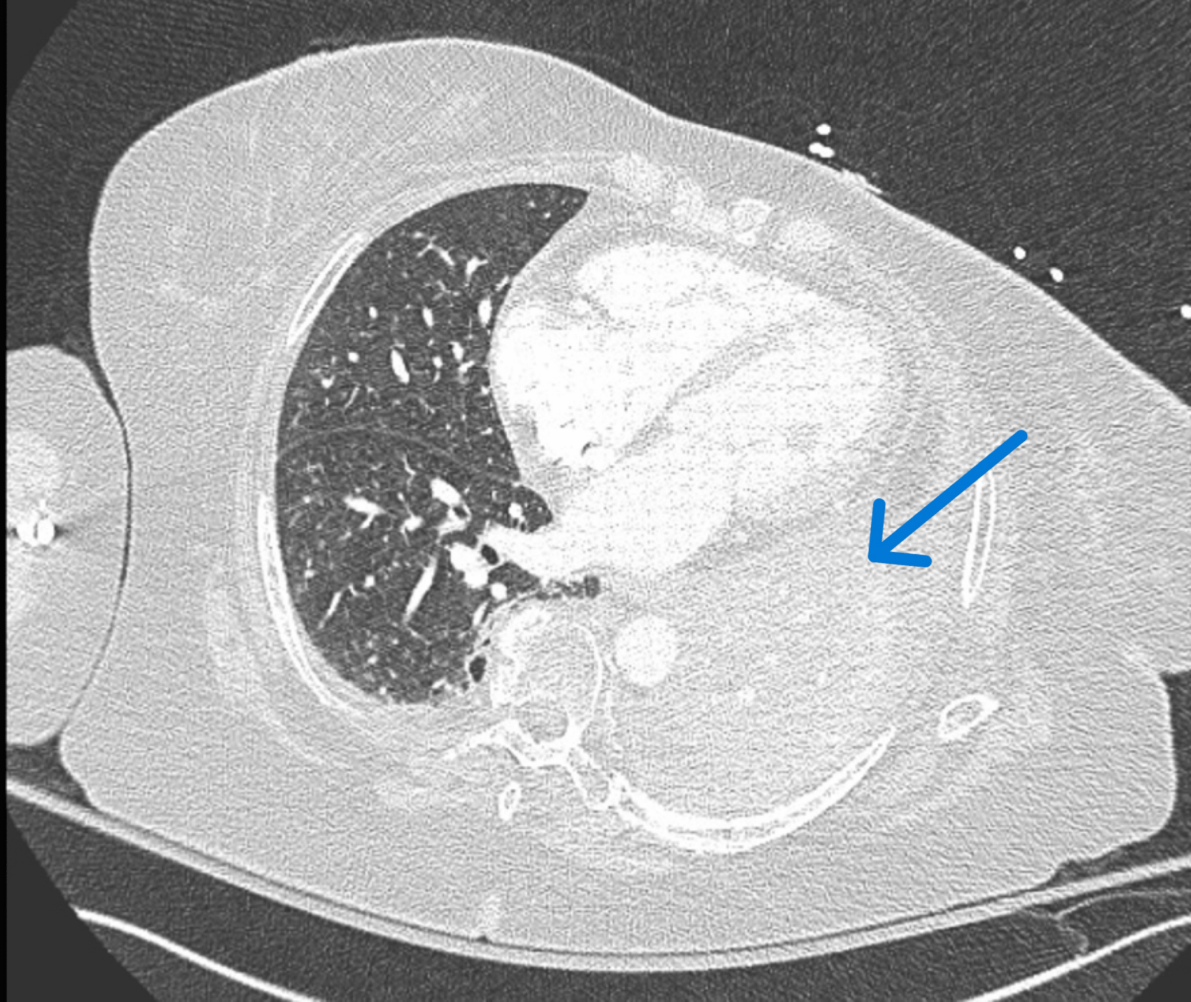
CT PE on Day 1 of Presentation There was no evidence of acute PE. There was stable complete occlusion of L mainstem bronchus and bronchi (blue arrow). PE, pulmonary embolism.
